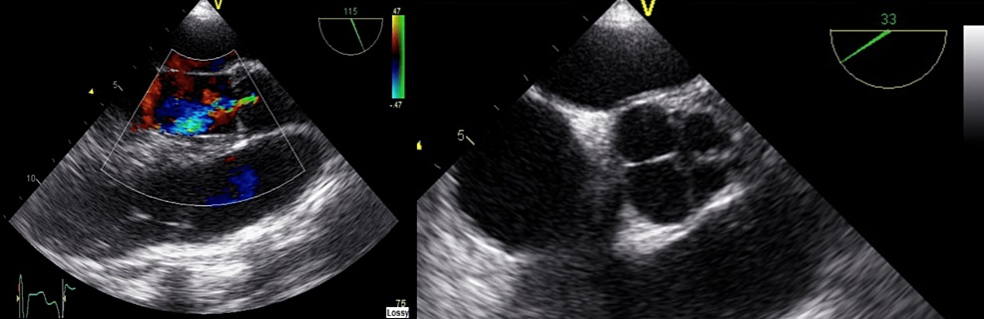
Aortic valve regurgitation on transesophageal echocardiogram (TEE) long axis aortic valve view (left) and quadricuspid aortic valve on TEE short axis aortic valve view (right).
